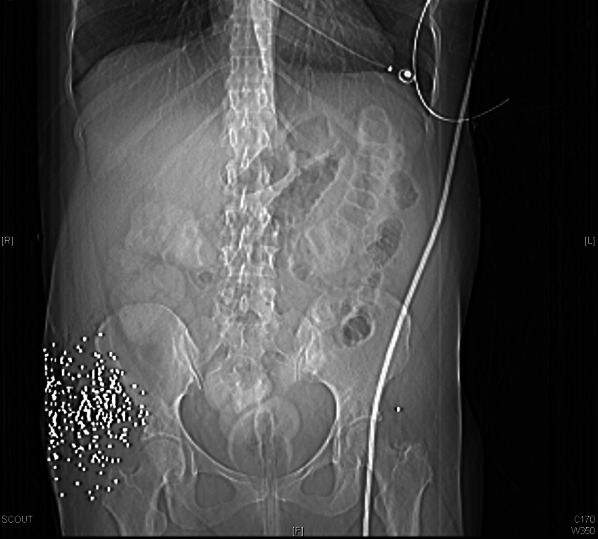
Abdominal X-ray showing numerous shot pellets contained in a relatively small area near the patient’s right hip
